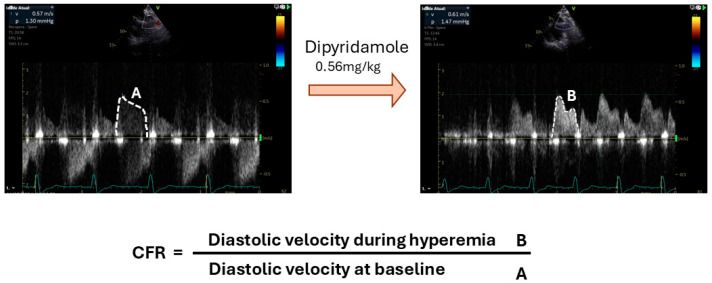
Echocardiography-Derived Coronary Flow Volume Reserve. Using transthoracic Doppler echocardiography with a pulsed-wave sample placed on the anterior descending artery, mean diastolic velocities are measured at rest and during stress to calculate coronary flow velocity reserve.
